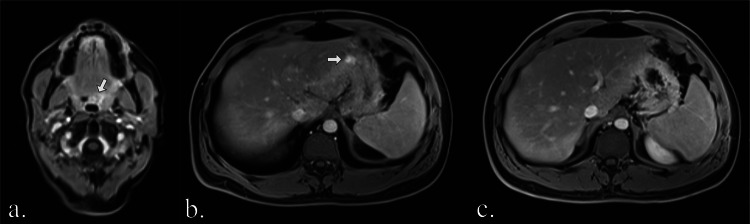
Examples of false positive findings: Contrast-enhancement in the T1 Dixon water-only image of the pharyngeal wall (white arrow in a) was confirmed as regular hyperplastic pharyngeal tissue on biopsy. A focal contrast-enhancement of the gastric wall (white arrow in b) was no longer detectable on follow-up MRI (c) and was retrospectively deemed an artifact. Images were cropped for better visualization
